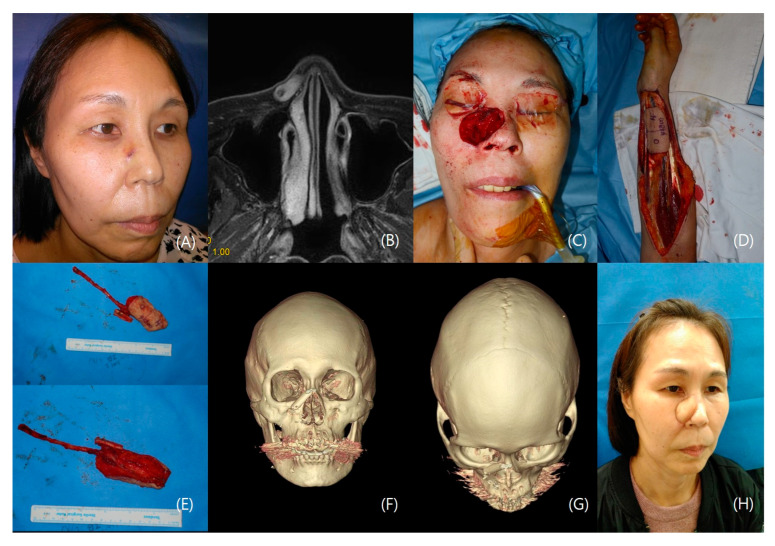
A case of a patient with basal cell carcinoma in the right nose. (A) Preoperative photo. (B) Pre-operative MRI (C) 5 × 4 cm defect including right nasal bone and nasal mucosal lining. (D) Design of the radial forearm flap, including the nasal mucosal lining (E) Radial forearm osteocutaneous flap harvested. (Distal radius included) (F) Post-operative 3D-CT AP view. (G) Post-operative 3D-CT Bird’s eye view. (H) Postoperative 5-month photo.
